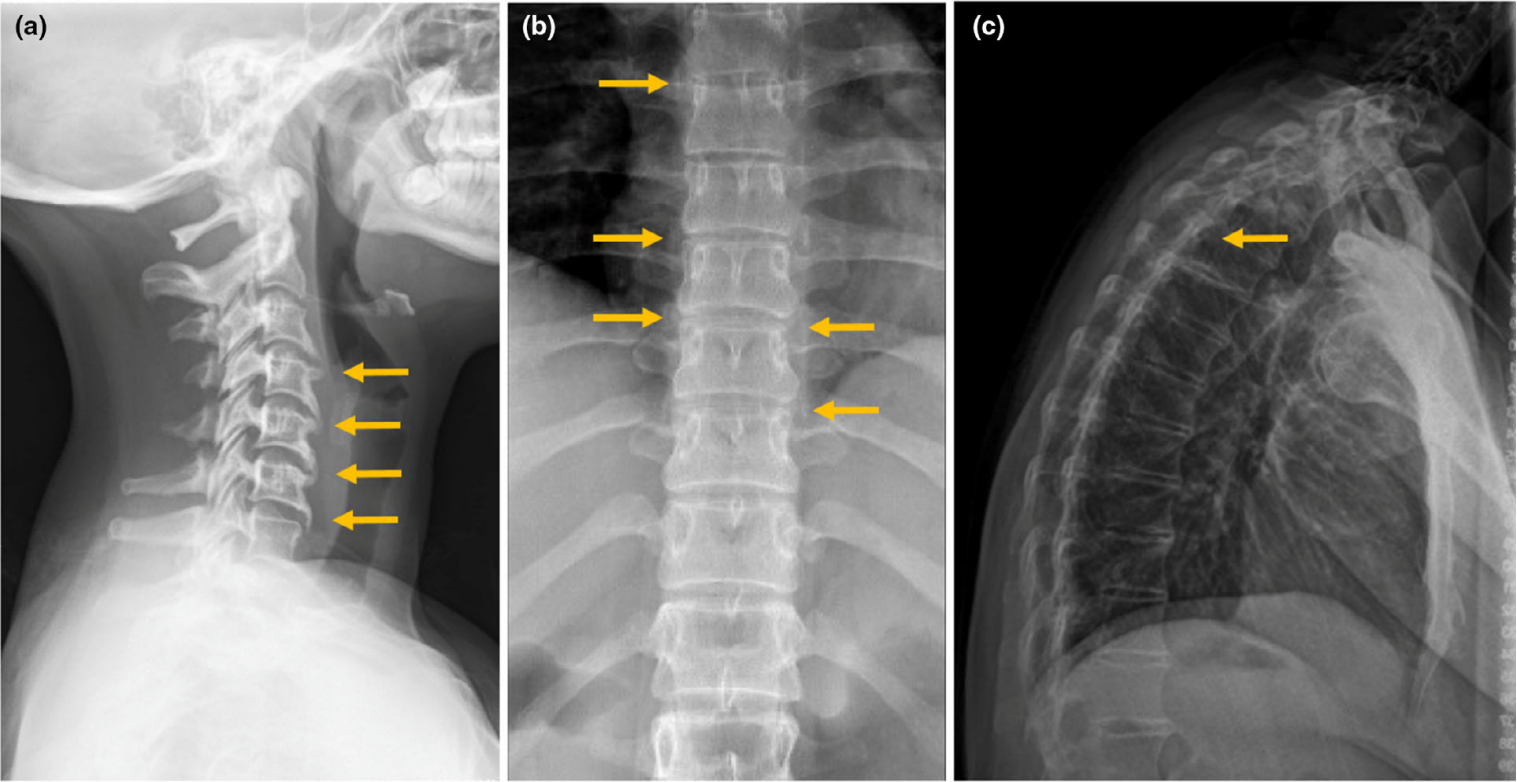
(a) A lateral cervical spine radiograph of a 25‐year‐old woman (Patient 1) who was treated with acitretin and isotretinoin for lamellar ichthyosis, showing radiographic features of straightening of the normal cervical lordosis and anterior ossification from C3 to C7 (orange arrows), with appearances in keeping with skeletal hyperostosis. (b) An anterior thoracic spine radiograph of a 38‐year‐old woman (Patient 2) who was treated with isotretinoin for Darier disease, showing radiographic features of bridging osteophytes seen bilaterally (orange arrows) and anteriorly through the thoracic spine. (c) A lateral thoracolumbar spine radiograph of a 43‐year‐old woman (Patient 3) who was treated with isotretinoin for epidermolytic ichthyosis, with radiographic features showing diffuse syndesmophytosis with ossification of the anterior longitudinal ligament, with a linear longitudinal radiodense stripe also running centrally along the vertebral column (yellow arrow) in keeping with ossification of the interspinous/supraspinous ligaments with loss of the normal lumbar lordosis and in keeping with diffuse hyperostosis.
